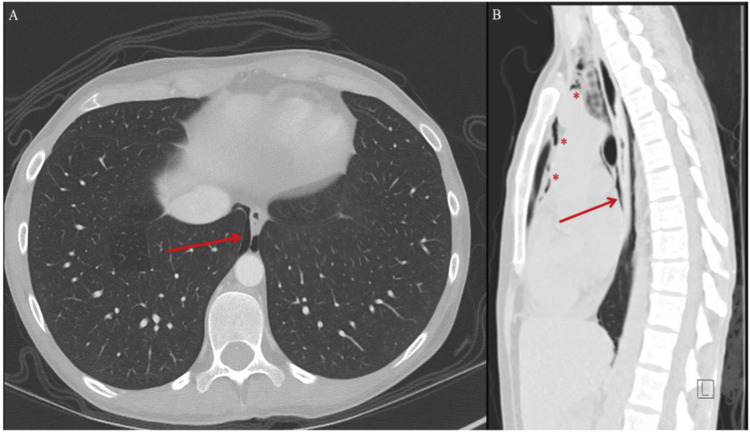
Computed tomography (CT) of the chest in axial (panel A) and sagittal (panel B) views obtained without the administration of oral or intravenous contrast material showing nonspecific pneumomediastinum, with contained air seen surrounding the lower esophagus (panels A and B, arrows). Free air is also seen extending along the anterior and superior mediastinum (panel B, asterisks).
